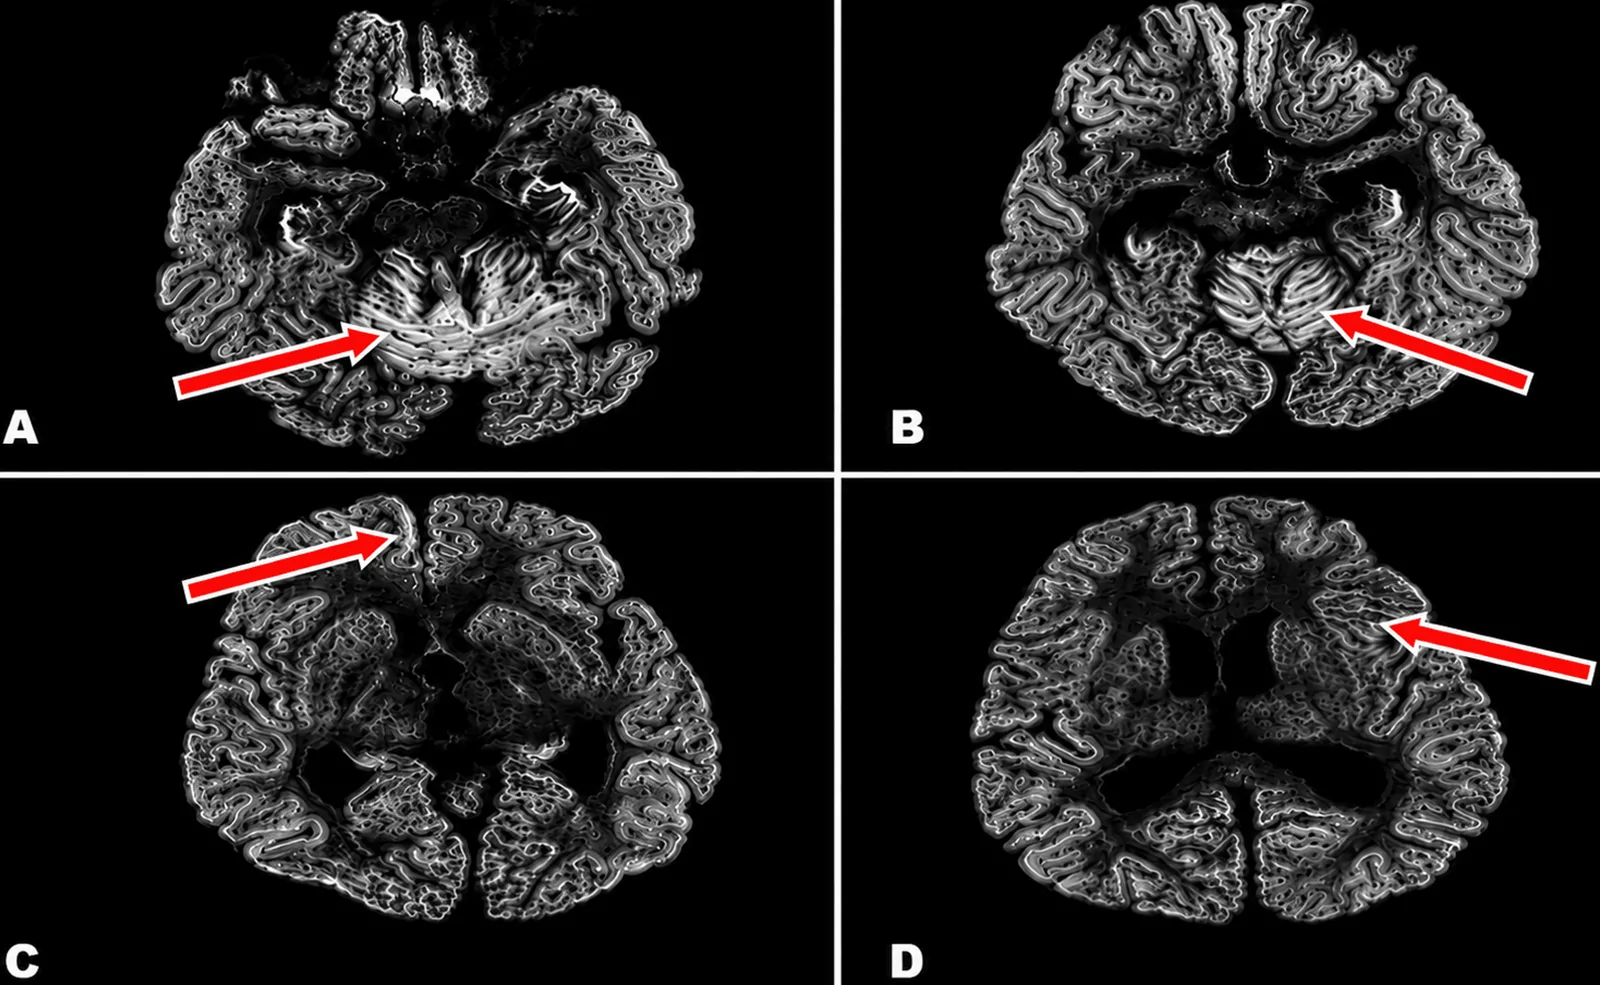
Axial brain MRI at different levels demonstrating hypoxic-ischemic encephalopathy (A) Cerebellar cortex. (B) Thalami and deep gray nuclei. (C) Frontal cortex. (D) Parietal cortex. Arrows indicate the areas of abnormal signal intensity.
